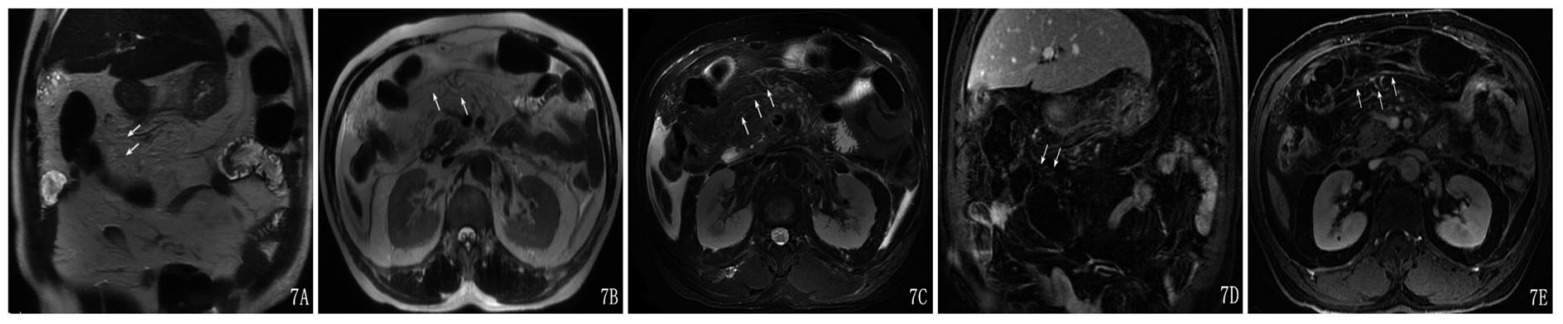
A 46-year-old male with mild AP and TMI was Grade I. Transverse-mesocolon-vessel involvement could be seen on a coronal SSFSE T2-weighted image (A), on an axial SSFSE T2-weighted image (B) and on an axial SSFSE T2-weighted image with fat suppression (C). The transverse mesocolon vessels showed the vessel-lumen expansion and dilated and tortuous lateral-branch small vessels on enhancement of the coronal image (D) and axial image (E).
